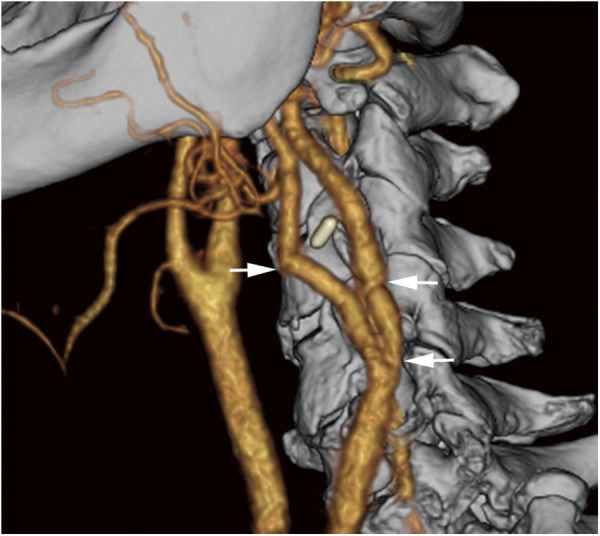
Fig. 3 A three-dimensional view of postoperative enhanced computed tomography findings obtained one year after the operation. The white arrows indicate the anastomosis site.
